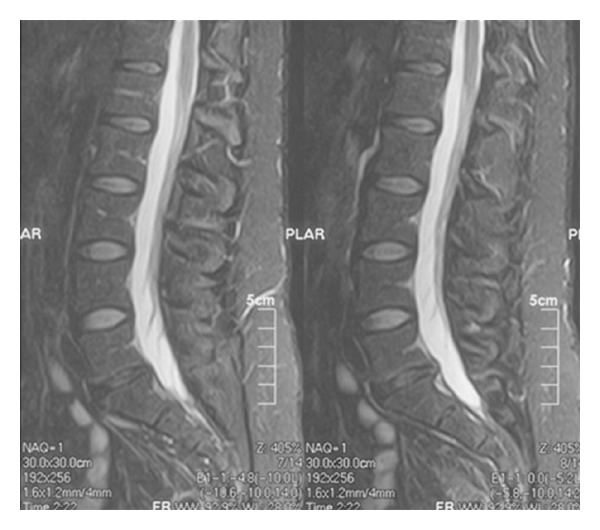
MRI sagittal T2-weighted demonstrating decreased disc signal at L5-S1. This indicates decreased hydration of the disc space. It does include or exclude patient symptoms of back pain.
